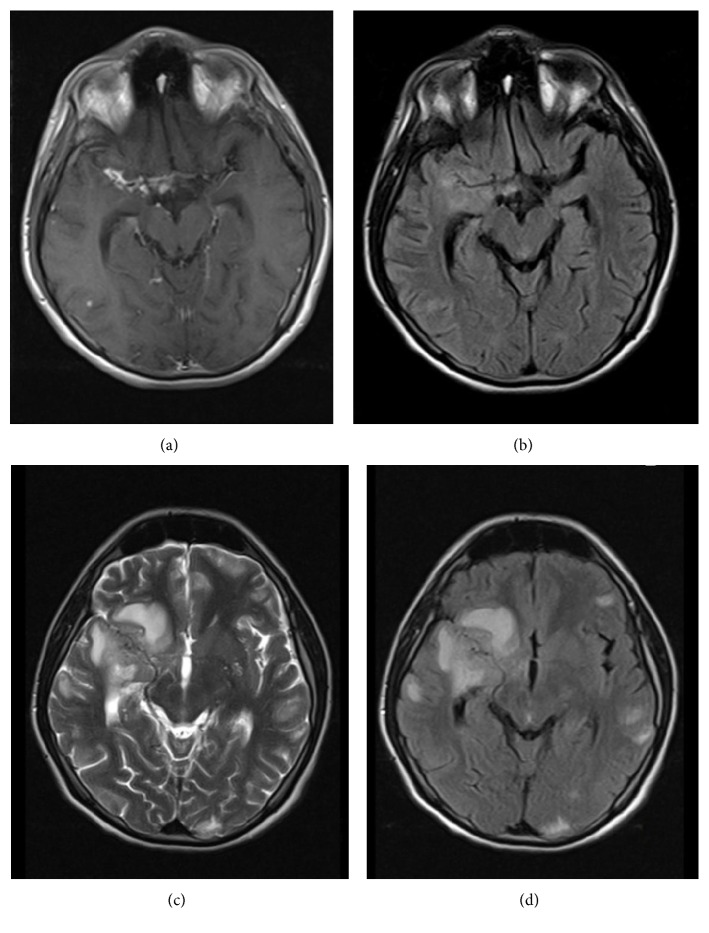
A contrast of an initial diagnosis against its progression after one month T1A (a), FLAIR (b), a diagnosis 45 days later with T2A (c), and FLAIR (d) images. A meningeal contrast is involved and a thickening compatible with a diagnosis of basal meningitis is visible in the neighborhood of the right sylvian fissure and basal cisterns (a) and increments of parenchymal T2 intensity in that region (b). Instead of antituberculosis treatments working, on the forty-fifth day mark, parenchymal lesions have been proven to grow and new lesions have appeared (c, d).
